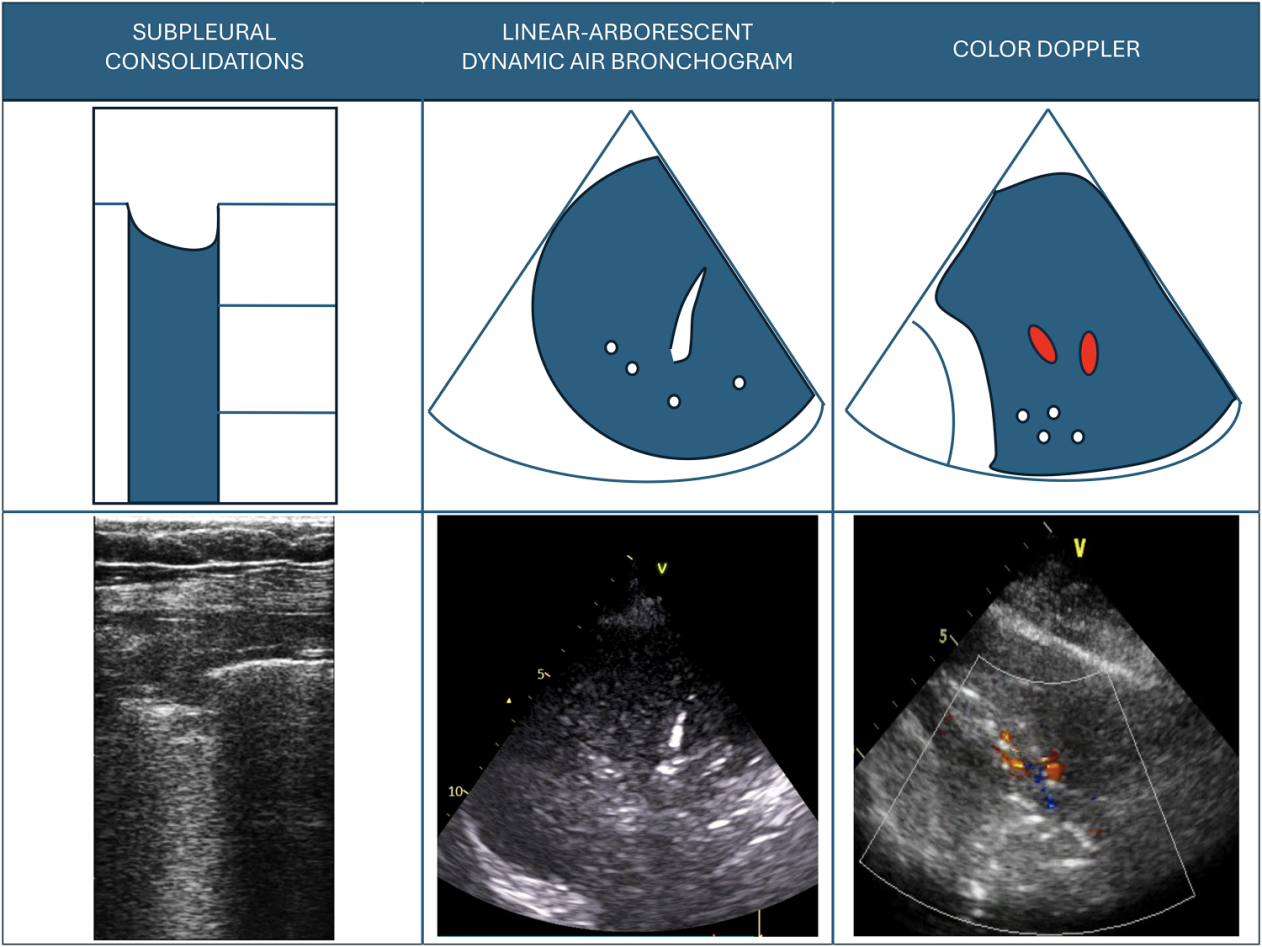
Ultrasound signs visualized in ventilator-associated pneumonia.
